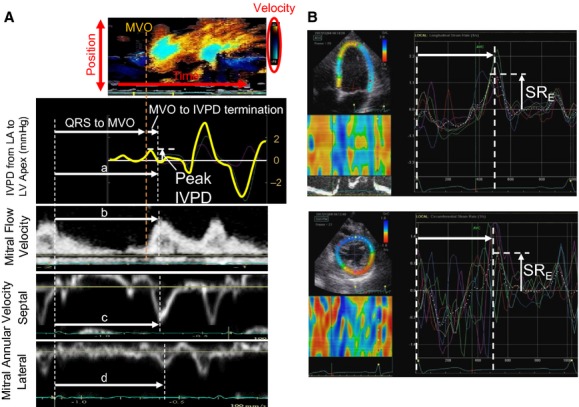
(A) The intraventricular pressure difference (IVPD) from the LA to the LV apex (second top panel) was extracted from the color M‐mode Doppler image (top panel) by integrating the Euler equation. The white vertical arrow indicates the early diastolic peak of the IVPD. The time from the peak QRS wave to the termination of IVPD (a), to the peak E wave (b), and peak septal (c) and lateral e′ (d) were measured. (B) The longitudinal strain rate (SR) (above) and circumferential SR (below) curves are displayed. The colored lines indicate segmental SRs and the white dashed curves indicate global SR. The early diastolic peak of global SR (SR_E_) and time from QRS to SR_E_ (white horizontal arrows) were measured. LA, left atrium; LV, left ventricular; E, early diastolic mitral flow; e′, early diastolic mitral annular velocity; MVO, mitral valve opening.
